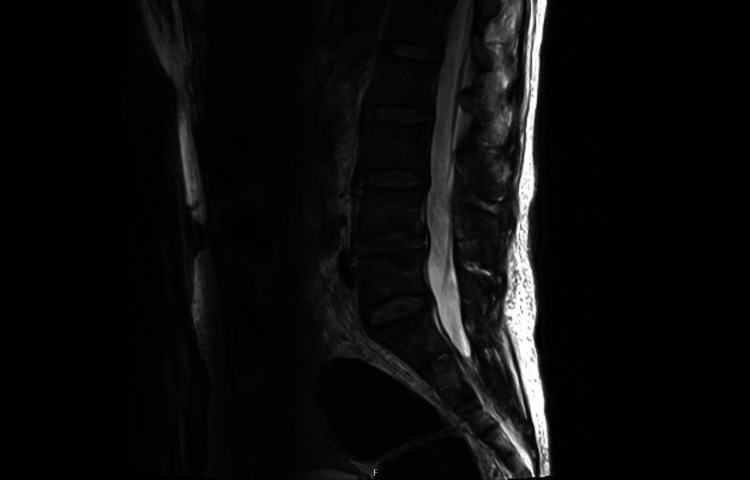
Lumbosacral spine MRI showing no secondary infiltration or radicular involvement (Case 2) MRI: magnetic resonance imaging
